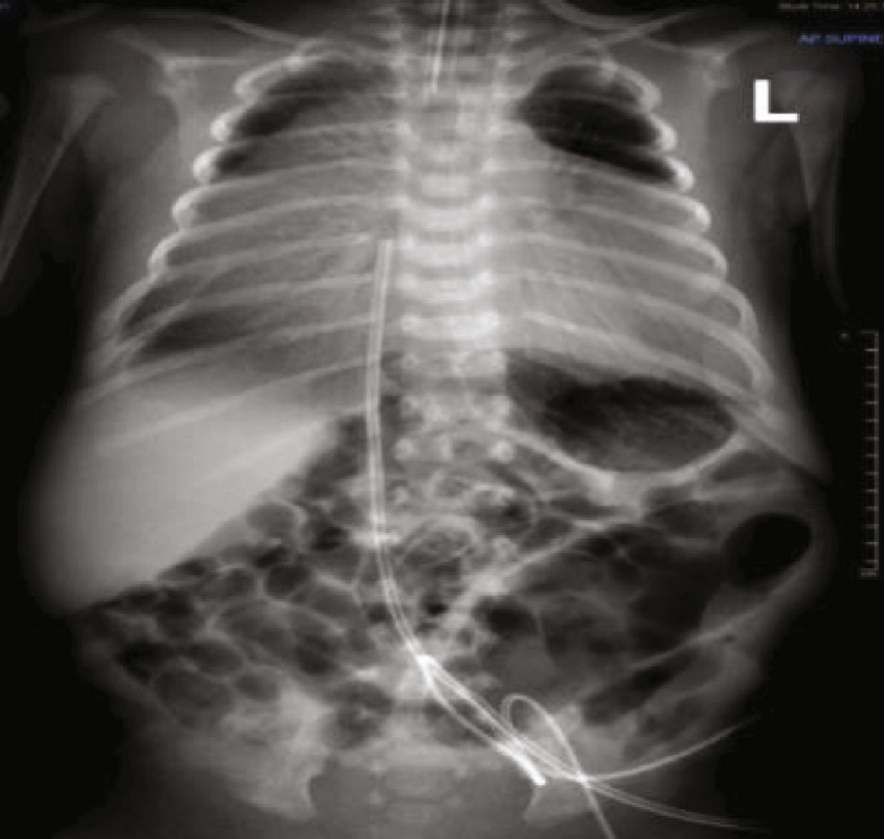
The initial chest and abdominal radiograph, showing a small lung volume, a large cardiothymic shadow, and air bronchogram streaks indicative of neonatal surfactant deficiency syndrome.
